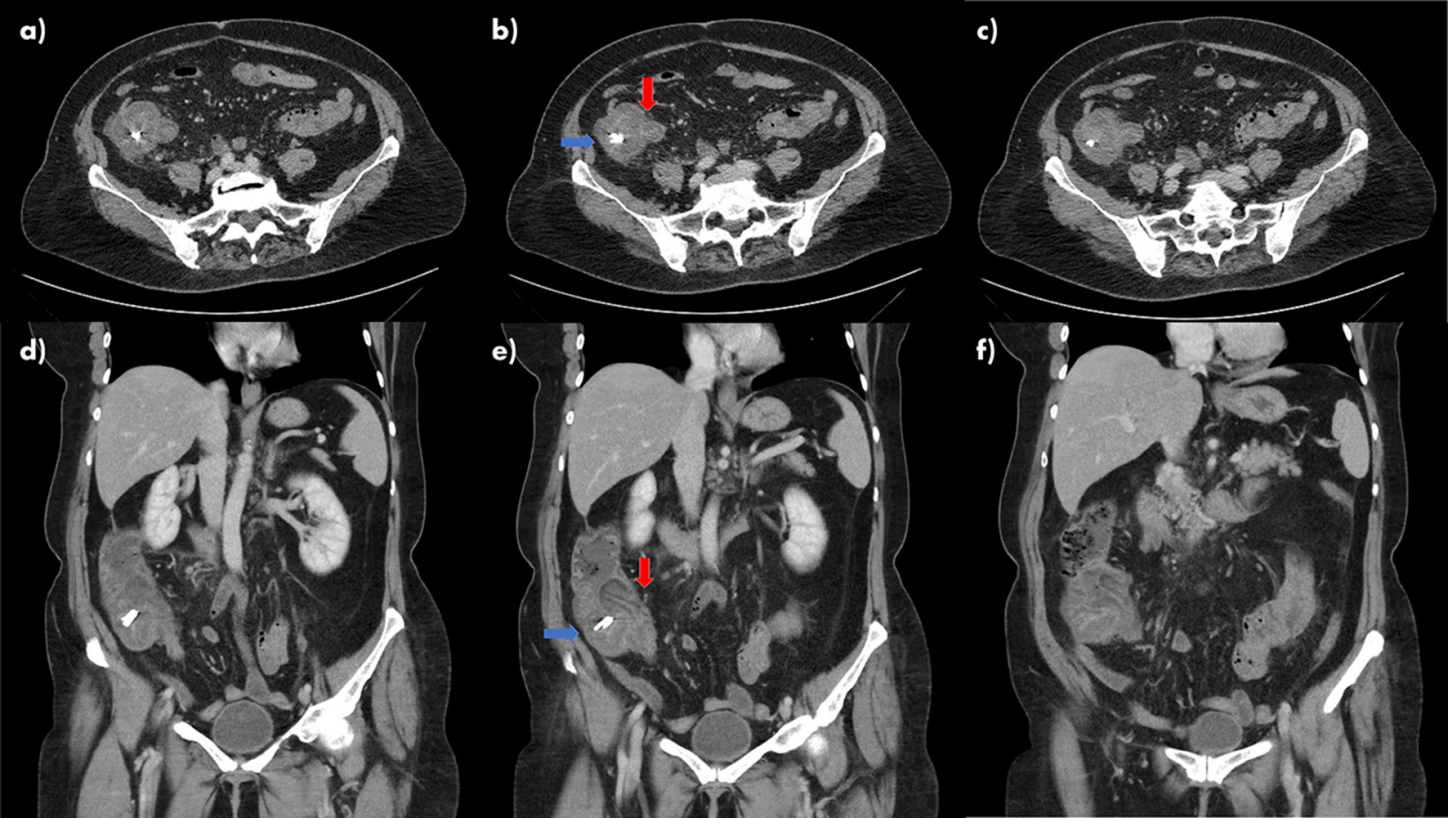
(a–f) Biphasic contrast-enhanced CT scan of the abdomen and pelvis demonstrating the "pseudodonut" sign as a result of thickening of ileocaecal wall due to post-polypectomy syndrome in successive axial (a–c) and coronal (d–f) images. Blue arrows (b, e) show caecum containing radiopaque clips applied during colonoscopy. Red arrows (b, e) show the ileocaecal valve. The thickening of the terminal ileum gives the impression of the donut sign seen in intussusception.
